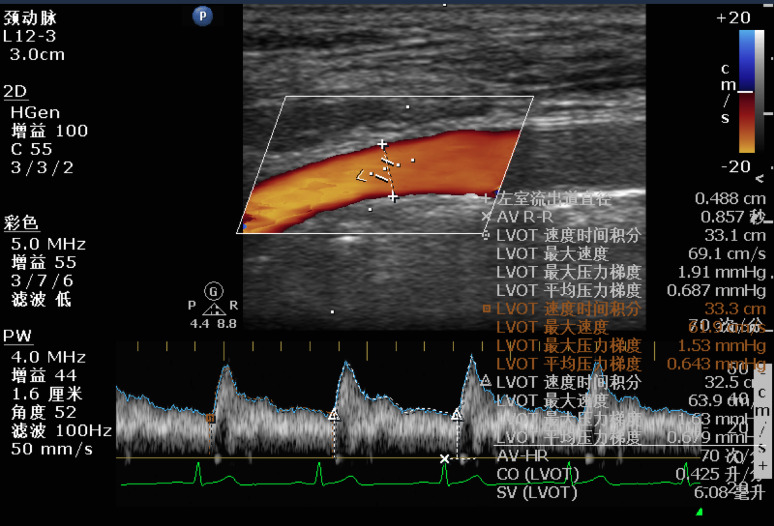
Ultrasound Image of ICA-VTI. ICA= Internal Carotid Artery; VTI= Velocity Time Integral
